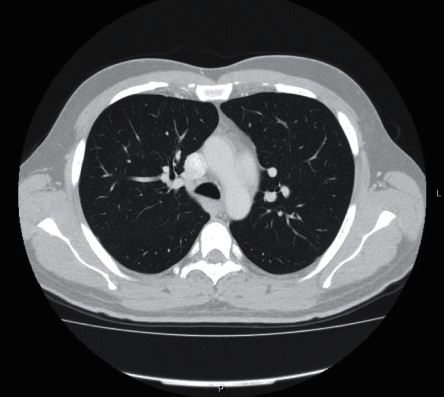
Follow-up chest CT showing resolution of the ground glass areas and the bilateral pleural effusion.
